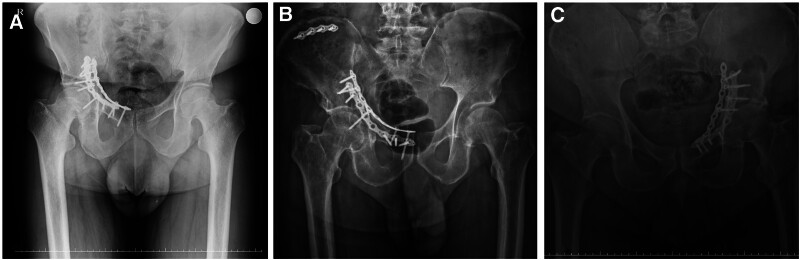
(A) X-ray image presenting the anatomic result of a patient at postoperative 12-month. (B) X-ray image presenting the good result of a patient at postoperative 12-month. (C) X-ray image presenting the poor result of a patient at postoperative 12-month.
